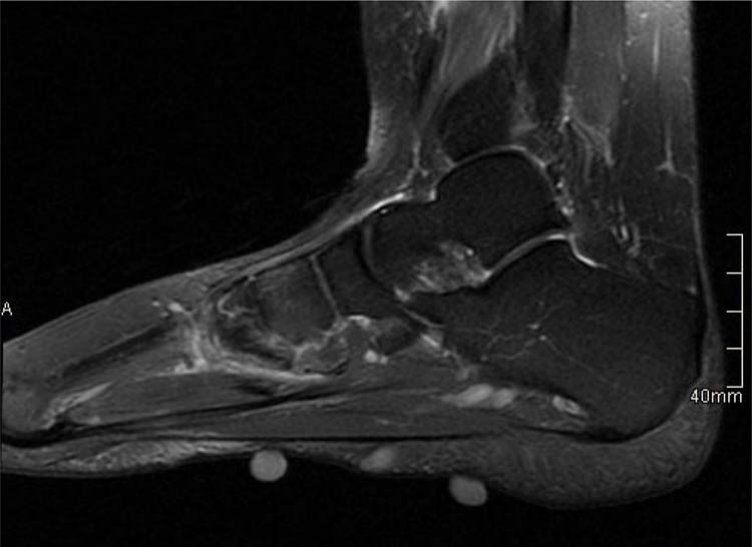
MRI with contrast; right foot—sagittal view.
